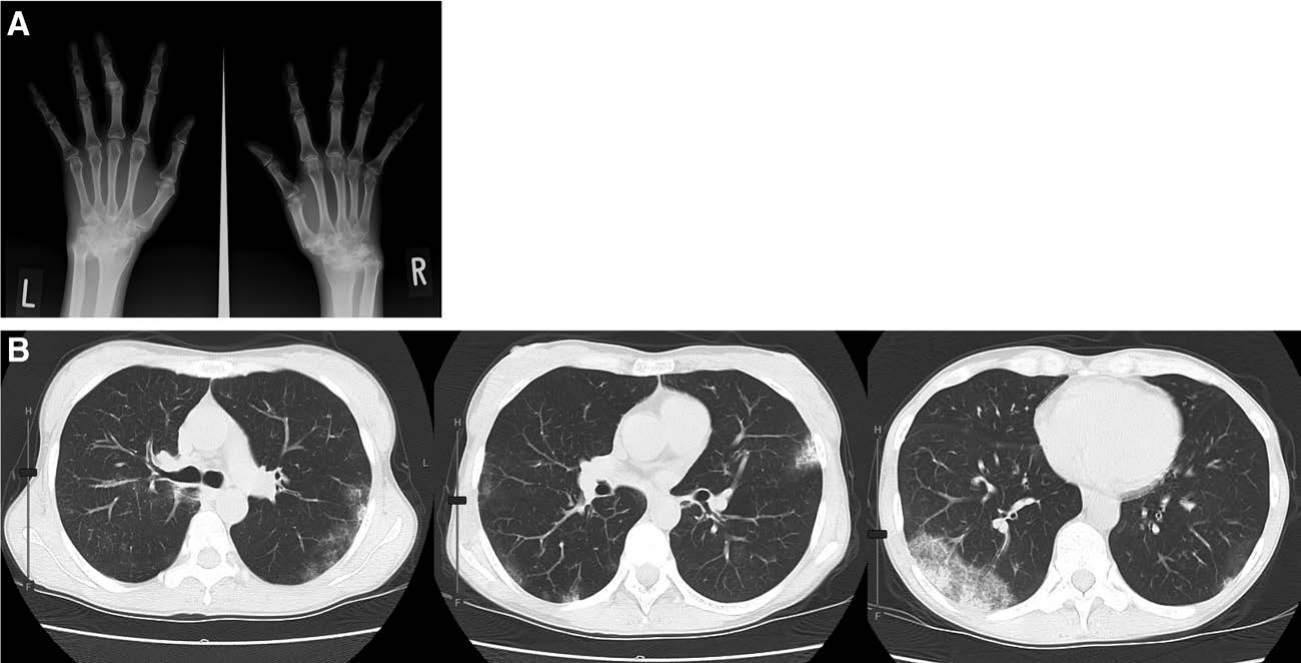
(A) X-ray of both hands showing loss of intercarpal and carpometacarpal joint spaces, ulna deviation of MCP joints, and deformity of the PIP joint of the left middle finger. (B) Chest computed tomography showing bilateral ground-glass opacities, similar to initial findings. MCP = metacarpophalangeal, PIP = proximal interphalangeal.
